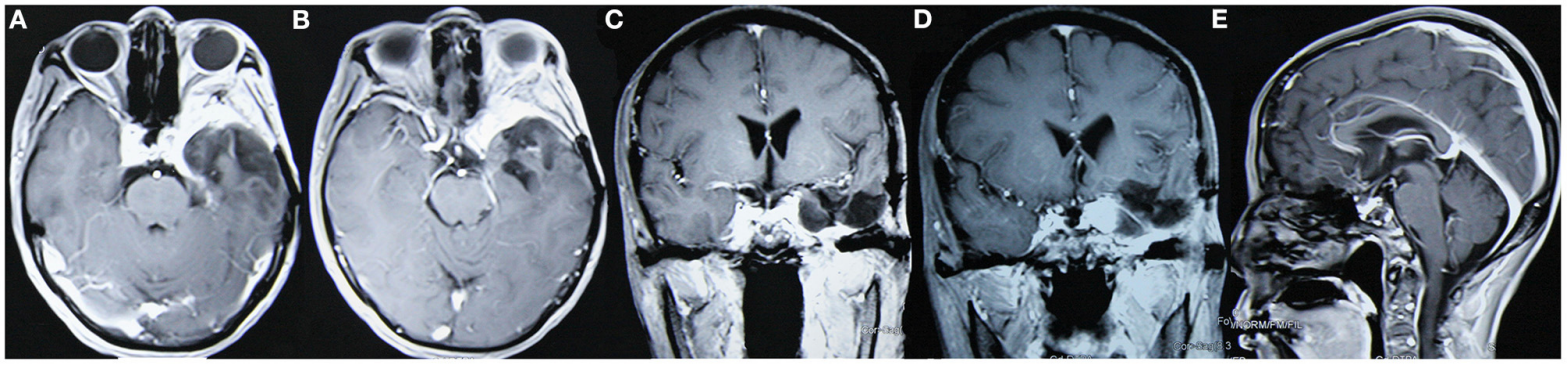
The tumor did not relapse as suggested in enhanced MRI reexamination 3 years after surgery. (A, B) Enhanced axial images show no tumor relapse in the central skull base; (C, D) enhanced coronal images show no tumor relapse in the cavernous sinus. (E) The enhanced sagittal image shows no tumor relapse in the anterior skull base.
